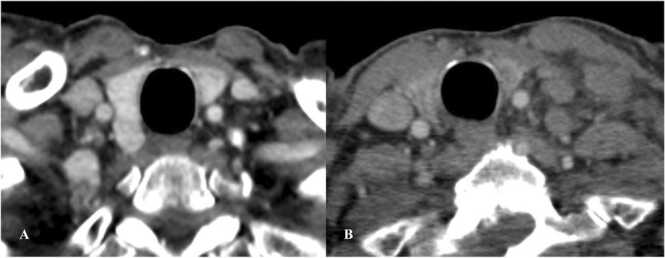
Immune-mediated thyroiditis in a 65-year-old man with lung cancer on Nivolumab. Contrast-enhanced axial chest CT images show A. Normal appearance of the thyroid before starting Nivolumab. B. There is atrophy of the thyroid with decrease in the enhancement after starting Nivolumab consistent with thyroiditis.
